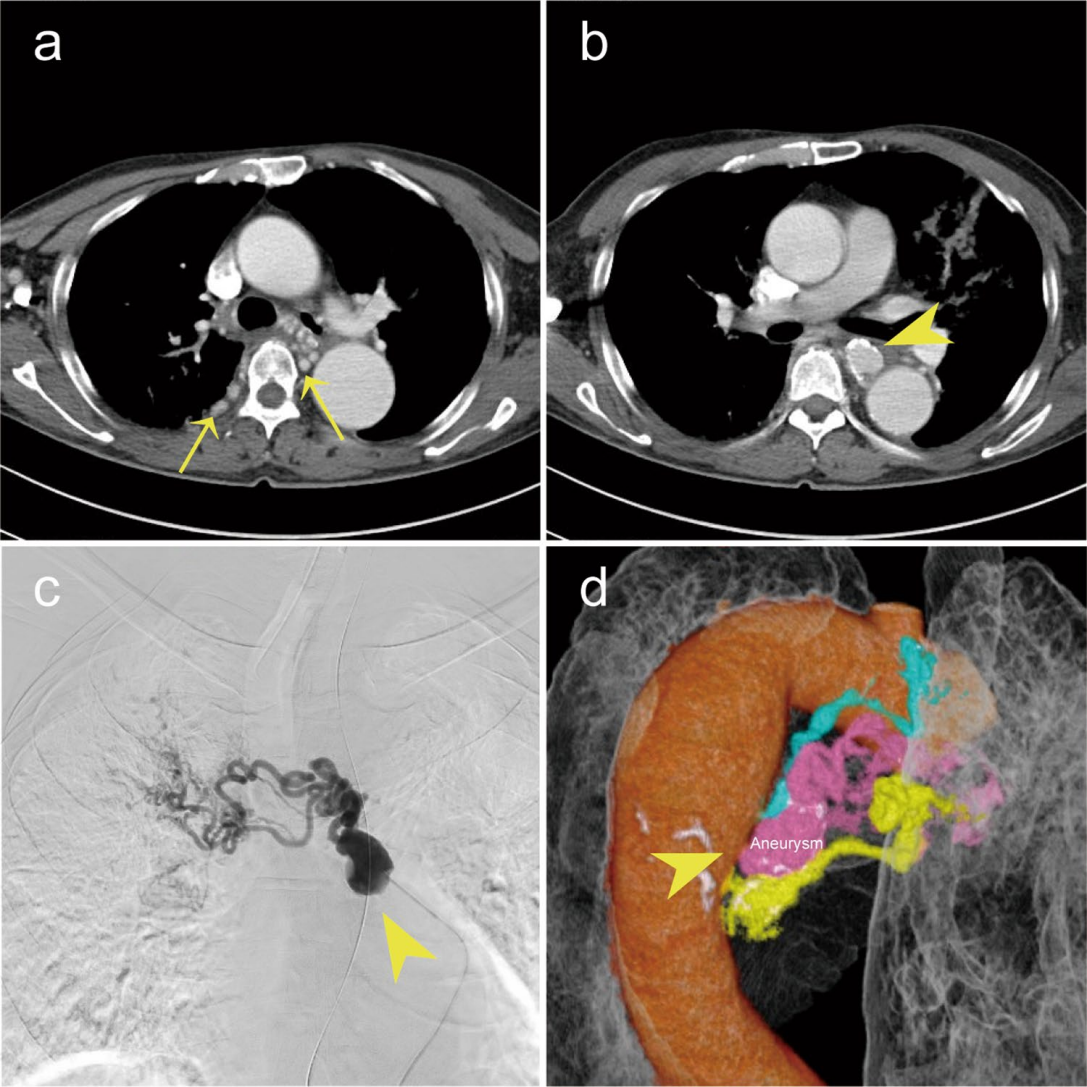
a Axial Contrast-enhanced CT of dilated bronchial and intercostal arteries (arrow). b Axial Contrast-enhanced CT of bronchial artery aneurysm (arrowhead). c Digital subtraction angiography of bronchial artery aneurysm (arrowhead) and dilated bronchial artery. d Contrast-enhanced CT image reconstructed in 3D and pseudo-colored. View from the back right of the patient, demonstrates no bronchial artery aneurysm (arrowhead)
